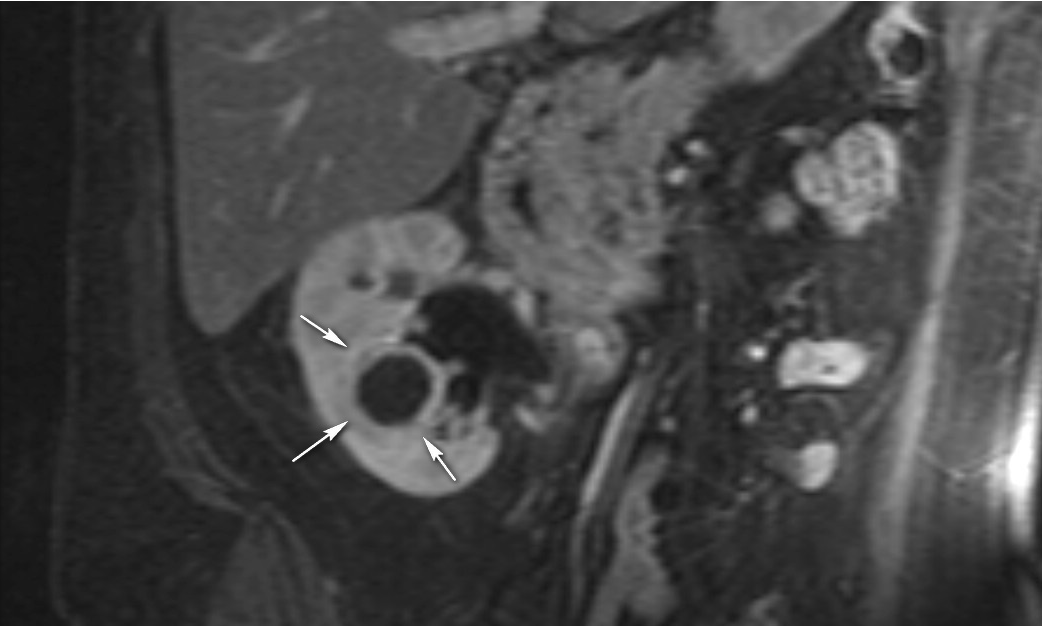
T1-weighted coronal magnetic resonance imaging scan with fat saturation and gadolinium enhancement. The cortical mass is seen as a low signal with an enhancing rim (arrows).
